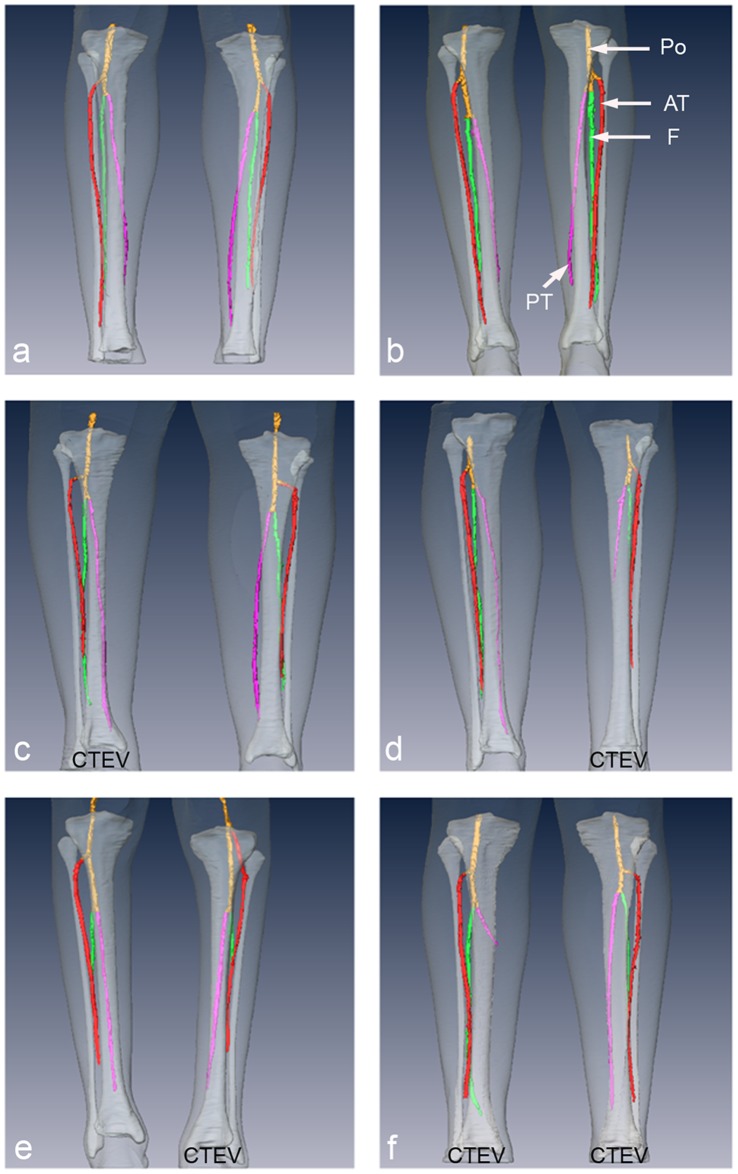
Anterior coronal 3D MRA reconstructions of arteries in legs of CTEV and control teenagers and young adults. The popliteral artery ‘Po’ (yellow), anterior tibia artery ‘AT’ (red), posterior tibia artery ‘PT’ (pink), and fibula artery ‘F’ (green) are overlaid onto 3D surface reconstructions of lower leg (transparent white) and tibia and fibula (white) from T1-weighted MRI. (a) C3, (b) C5, (c) U1, (d) U2, (e) U4, (f) B2.
